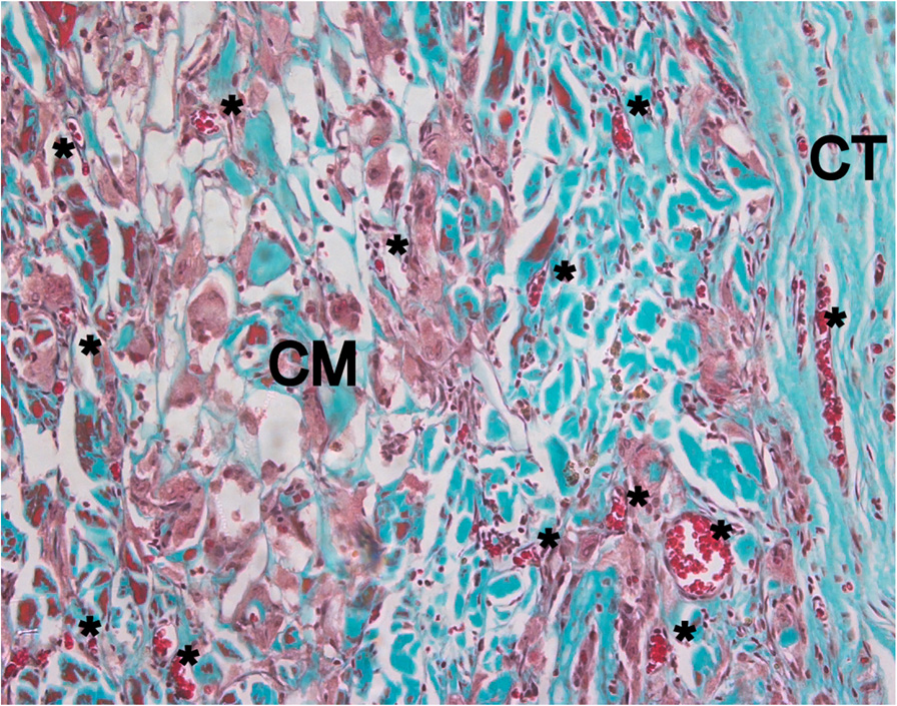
High number of multinucleated giant cells and advanced blood vessel invasion in the ECL matrix after 12 weeks healing period (Masson Goldner, orig. magn. 200×). CM: collagen matrix, MT: muscle tissue, *: blood vessel, CT: connective tissue.
